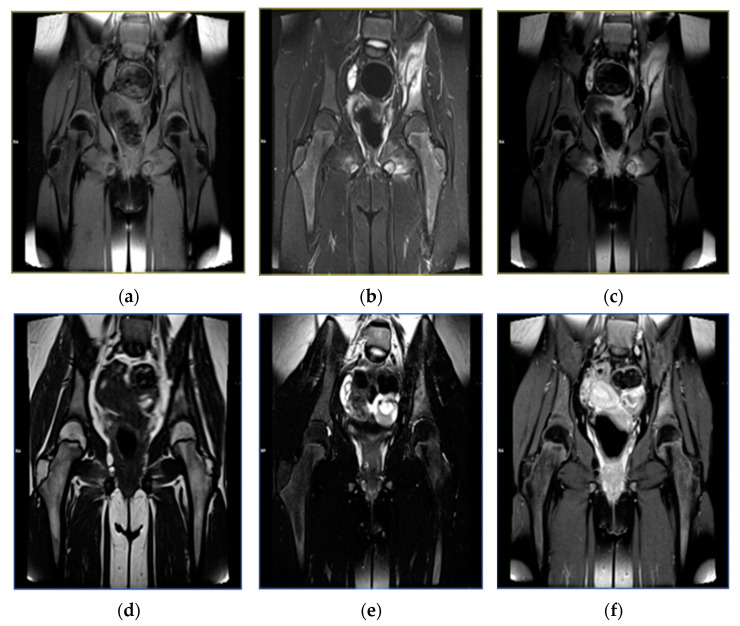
Magnetic resonance images in a 10-year-old female patient with inflammatory lesions around the left sacroiliac joint. Status before treatment: T1-weighted sequence (a), T2-weighted sequence (b), T1-weighted sequence with contrast enhancement (c). Status after a 19-month follow-up, with partial regression of the inflammatory lesions: T1-weighted sequence (d), T2-weighted sequence (e), T1-weighted sequence with contrast enhancement (f).
